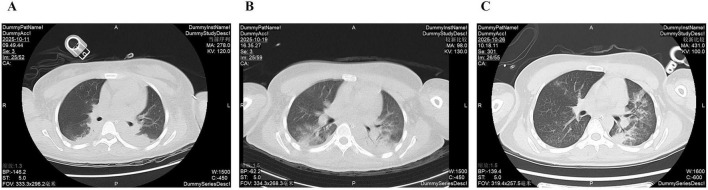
Chest CT images of the patient’s on Day 11 (A), Day 19 (B), Day 26 (C).
